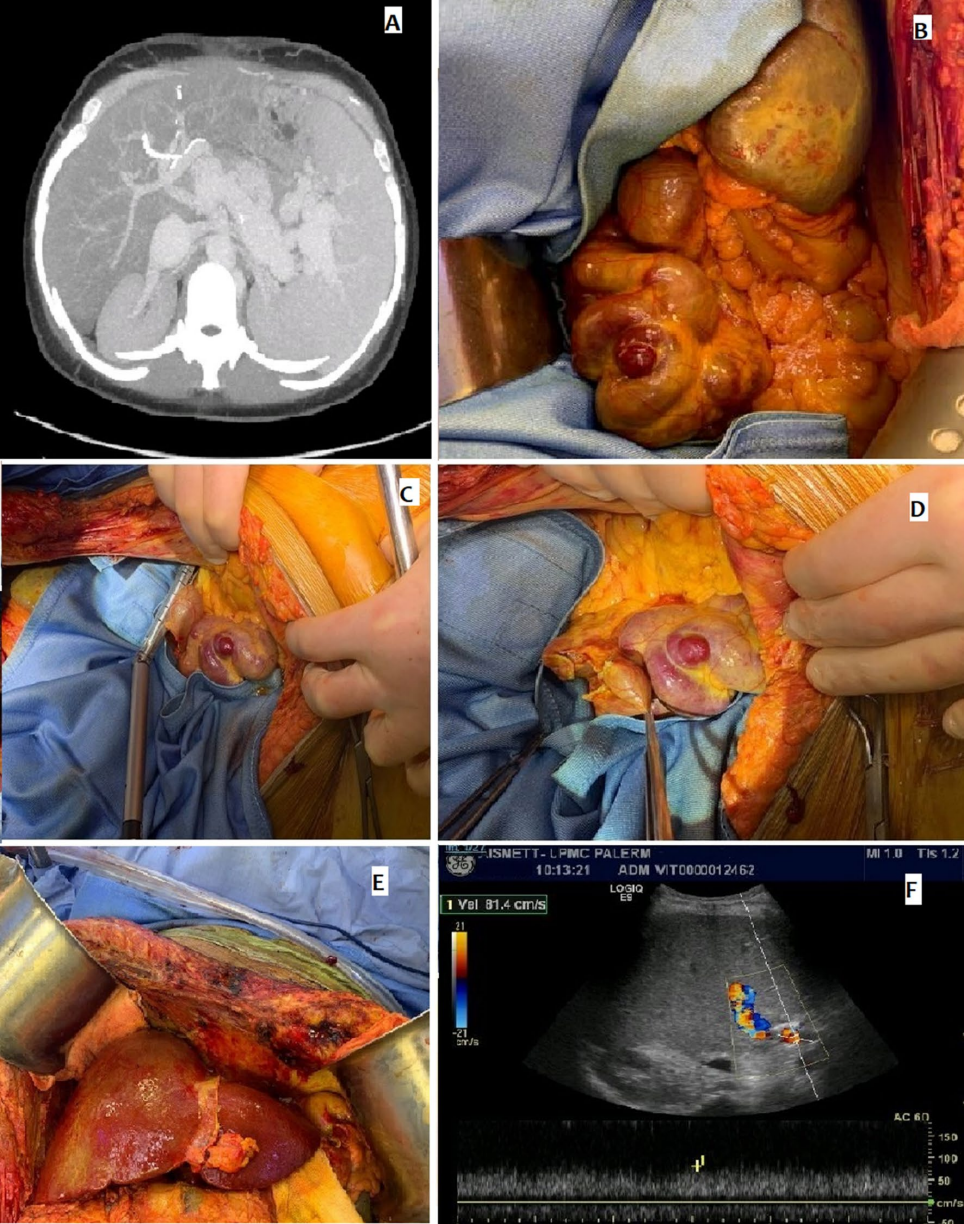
Intraoperative findings—giant spleno-renal shunt: A Tomographic image of splenocaval shunt; B Intraoperative findings of giant splenocaval shunt; C, D Stapler resection of the shunt; E Third liver after reperfusion; F Postoperative Doppler US
